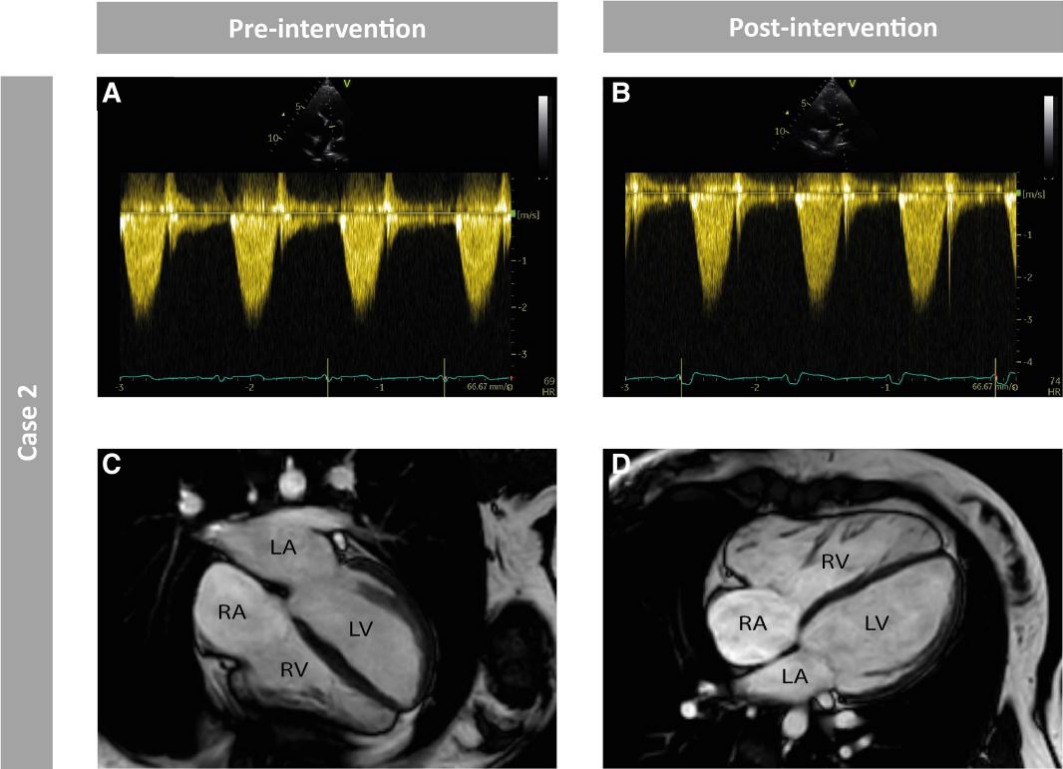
Pre- and post-interventional imaging of Case 2. *Figure 4* shows the pre- and post-interventional imaging of Case 2 using transthoracic echocardiography and cardiac magnetic resonance imaging. (*A*) Pre-interventional peak gradient of the right ventricular outflow tract (RVOT) measured using continuous wave Doppler echocardiography. (*B*) Post-interventional peak gradient of the RVOT measured using continuous wave Doppler echocardiography. Note the different scales in the vertical axis in panels *A* and *B*. (*C*) Pre-interventional four-chamber cardiac magnetic resonance (CMR) cine image. (*D*) Pre-interventional four-chamber CMR cine image. *A*–*B* show no decrease in post-interventional RVOT peak gradient compared with pre-interventional RVOT peak gradient using continuous wave Doppler echocardiography. *C*–*D* show pre- and post-interventional RV dilatation. Right ventricular systolic function was found to be reduced during CMR.
